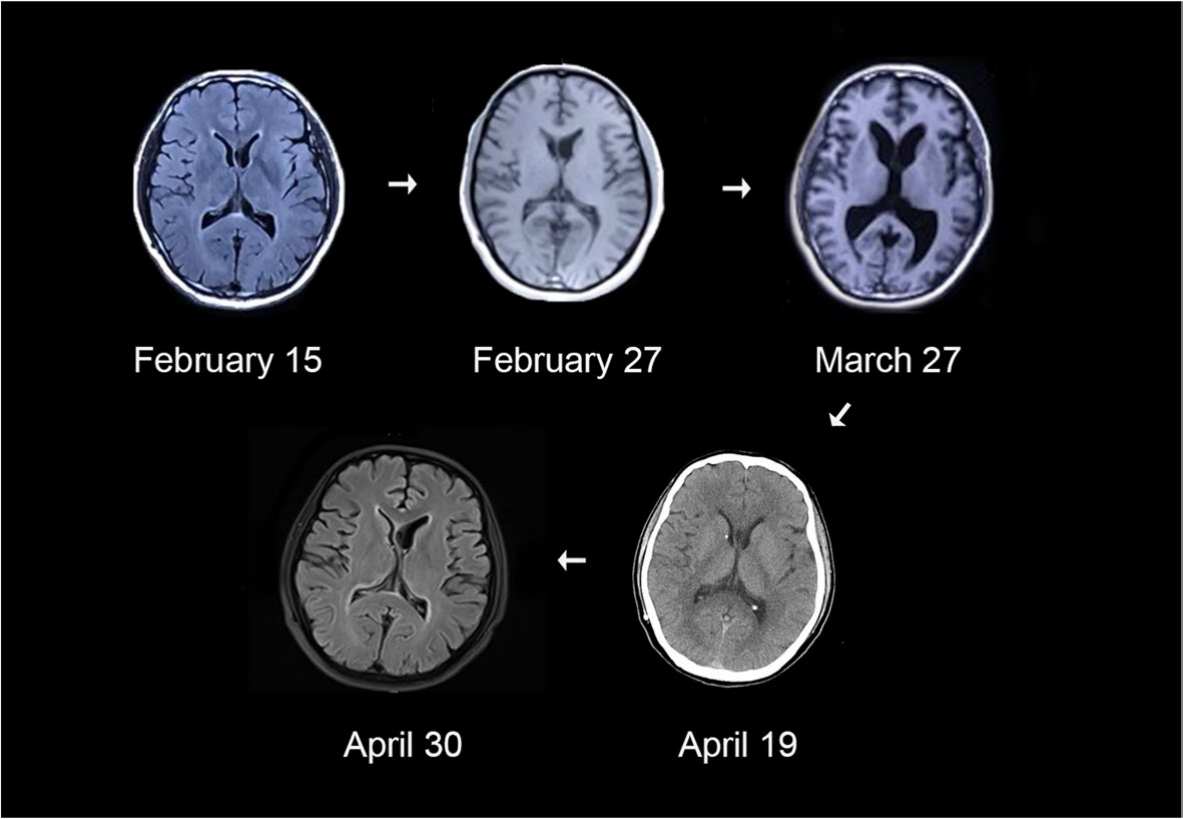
Serial axial MRI and CT scans showing that the ventricles changed significantly from normal to enlarged and then back to normal, showing the fluctuation of the lateral ventricle
